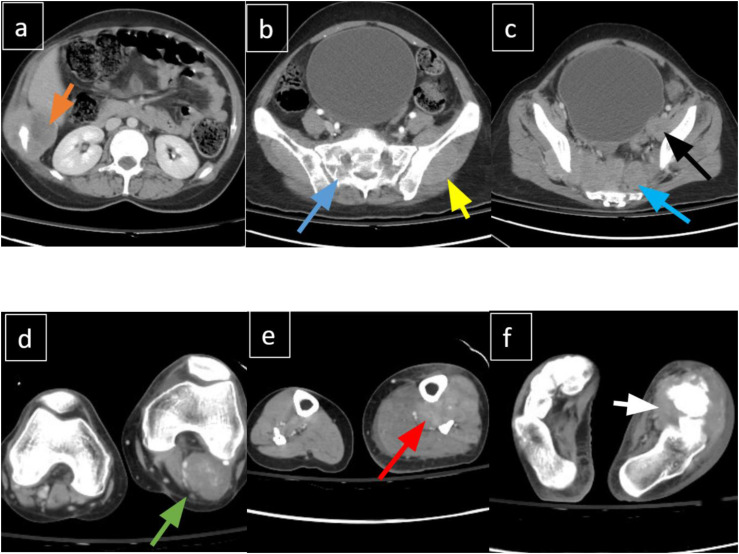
(A-F). Contrast-enhanced CT demonstrating widespread metastatic disease. (A) Right rib lesion with soft tissue component. (B and C) Pre-sacral mass with sacral infiltration, left iliac lesion, and local lymphadenopathy. (D-F) Enhancing soft tissue masses in the left popliteal fossa, lateral leg, and foot
